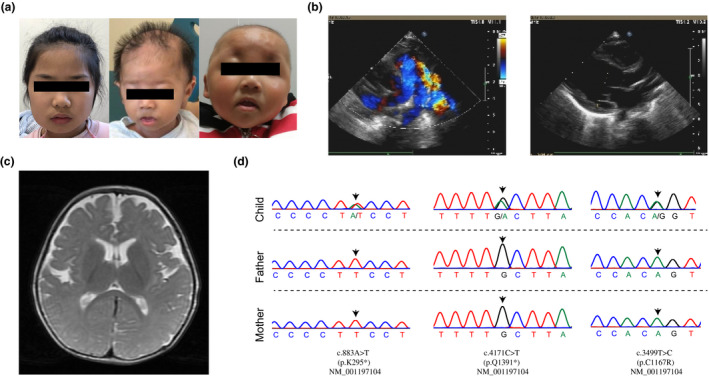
Clinical features and *KMT2A* variants of three WSS patients. (a) P1 had mild facial asymmetry, epicanthus tarsalis accompanied with severe ptosis of upper eyelid and small palpebral fissures, as well as astigmatism (left). P2 had sparse scalp hair, bilateral ptosis, mild hypertelorism, down‐slanting palpebral fissure, low‐set ears, long eyelashes, long philtrum, hypertrichosis, thin upper lip, and micrognathia (middle). P3 had prominent forehead, hypertelorism, wide nasal bridge, and low‐set ears (right). (b) Echocardiography indicated the likelihood of endocardial fibroelastosis, patent ductus arteriosus and patent foramen ovale in P2. (c) Brain magnetic resonance imaging revealed enlarged bilateral fissure and anterior longitudinal division. (d) Sanger sequencing validated three *de novo* variants in *KMT2A* in P1 (left), P2 (middle), and P3 (right).
